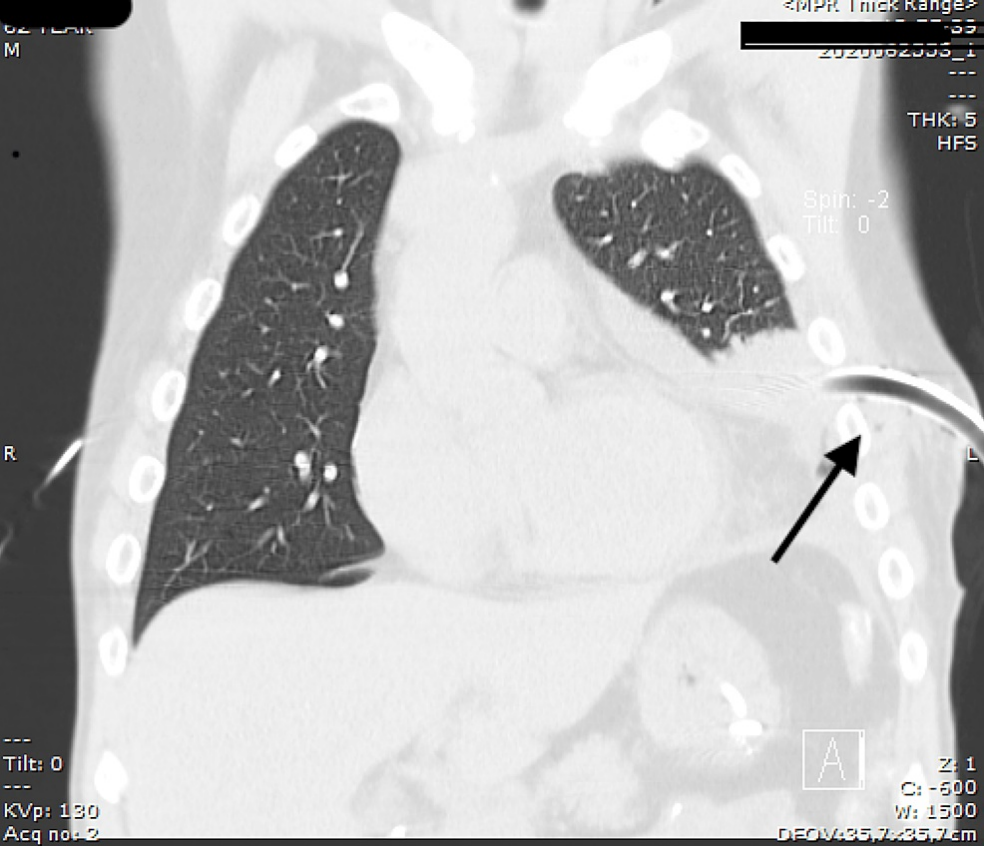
Coronal CT acquisitions showing the position of the first chest tube, placed in the fifth intercostal space (black arrow).
